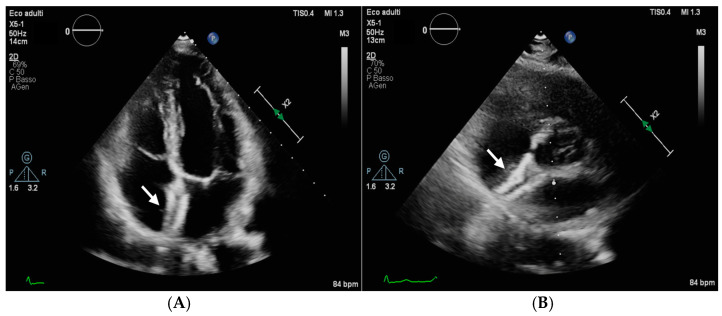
Six-month follow-up transthoracic echography of the patient who experienced late device embolization showing a well-positioned FFP device in a four-chamber view ((A), arrow) and in a parasternal short-axis view ((B), arrow).
